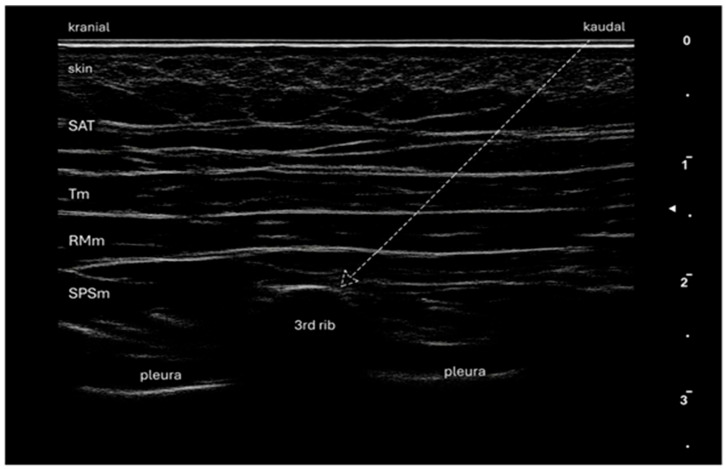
Ultrasound image demonstrating the serratus posterior superior intercostal plane block (SPSIPB). SAT: subcutaneous adipose tissue; Tm: trapezius muscle; RMm: rhomboid major muscle; SPSm: serratus posterior superior muscle. The dashed arrow indicates the path of the needle and its target point under ultrasound guidance.
